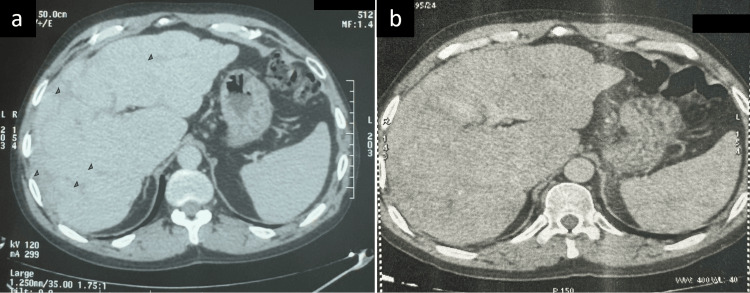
(a) Control abdominal CT scan at three months shows a significant reduction in hepatic nodules (arrows) and the disappearance of splenic nodules. (b) Control abdominal CT scan at 12 months shows regression of hepatic and splenic lesions.
